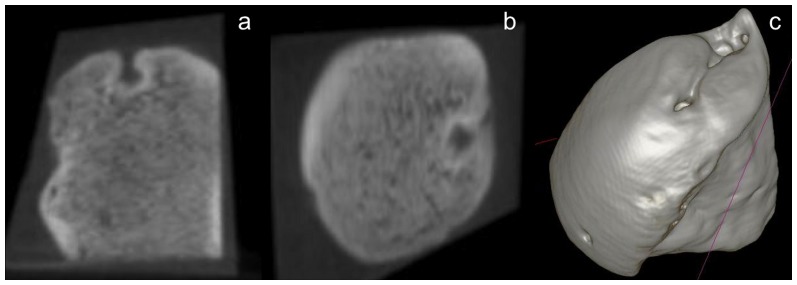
Cone-beam computed tomography. Cone-beam computed tomography of a representative medial femoral condyle delineated a cystic radiolucent area, which was smaller than the originally cylindrical repair area, indicating that the bone adjacent to the implanted scaffold began to remodel starting from the edges of the defect.
